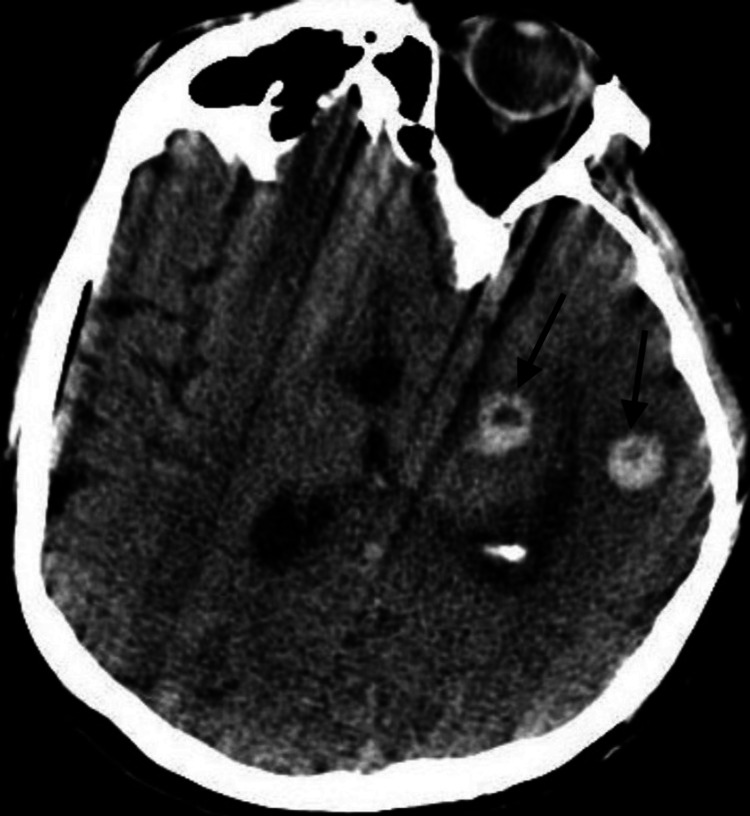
CT imaging without contrast revealed two ring-enhancing hyperdense lesions of 1.5 cm and 1.2 cm found in the left basal ganglia and left temporal lobe, respectively.
